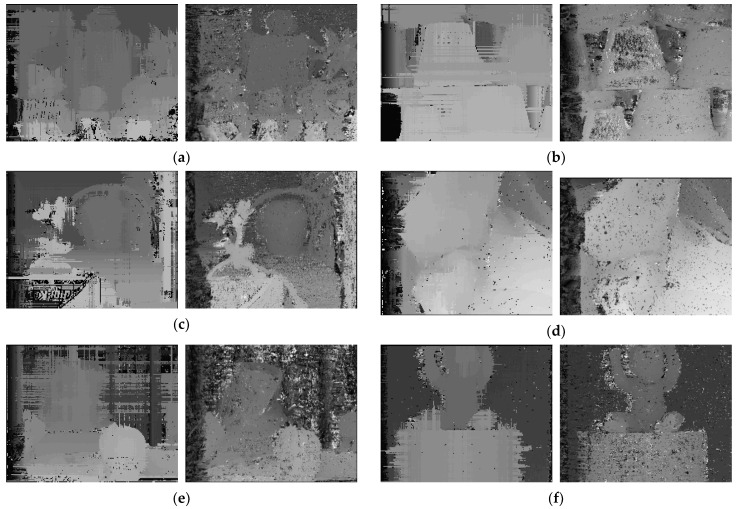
The results, sequentially, of CTHW and CT. (a) Moebius, (b) Flowerpots, (c) Reindeer, (d) Cloth2, (e) Midd1, and (f) Baby1.
